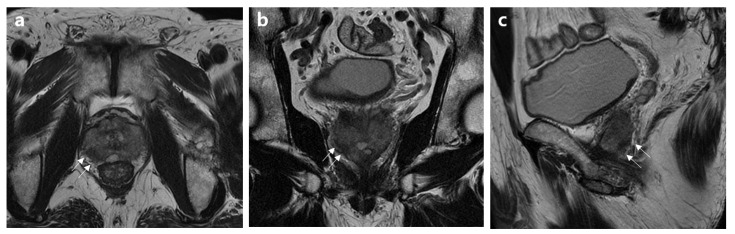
MR images of a 70-year-old patient without an extensive extraprostatic extension (EPE). (a) Axial, (b) coronal, and (c) sagittal T2-weighted MR images show capsular bulging or irregularity associated with a right peripheral zone mid-posterior lesion (arrows). The basic clinical findings included a serum PSA level of 15.8 ng/mL, a prostate volume of 34 mL, a positive biopsy core of 35%, clinical stage of T3a, biopsy grade 4, and high D’Amico risk. The PI-RADS v2.1 scores and Mehralivand EPE grades on multiparametric MRI were 5 and 2, respectively, according to both radiologists.
